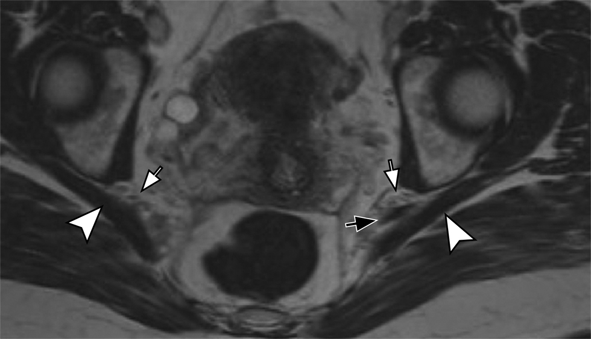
Fibrous band. A 41 year-old male with left sciatic nerve symptoms. Axial 3D volumetric T1-weighted image of the pelvis demonstrating both sciatic nerves (white arrows) and the piriformis muscles (arrowheads). There is a thick fibrous band (black arrow) posterior to the left sciatic nerve. (courtesy of Dr Denise Tokechi Amaral, São Paulo, Brazil).
